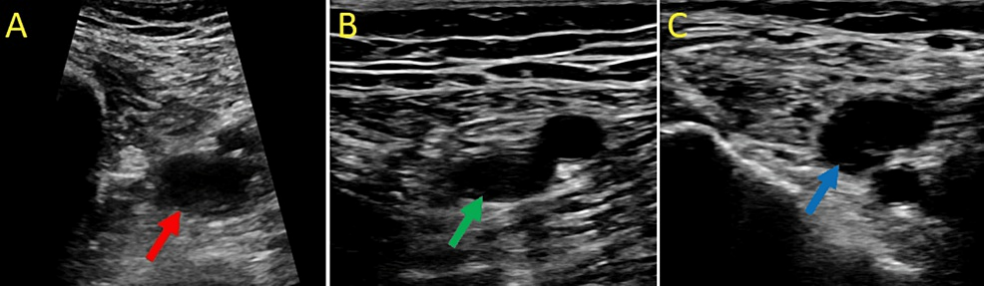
Ultrasound of the right lower extremity with incompressible veins with a transducer probe. (A) Non-compressible right proximal femoral vein (red arrow), (B) non-compressible right distal femoral vein (green arrow), and (C) non-compressible right popliteal vein (blue arrow). Non-compressible veins indicate the presence of venous thrombi, and these findings are consistent with DVT of the right lower extremity. DVT: deep vein thrombosis.
